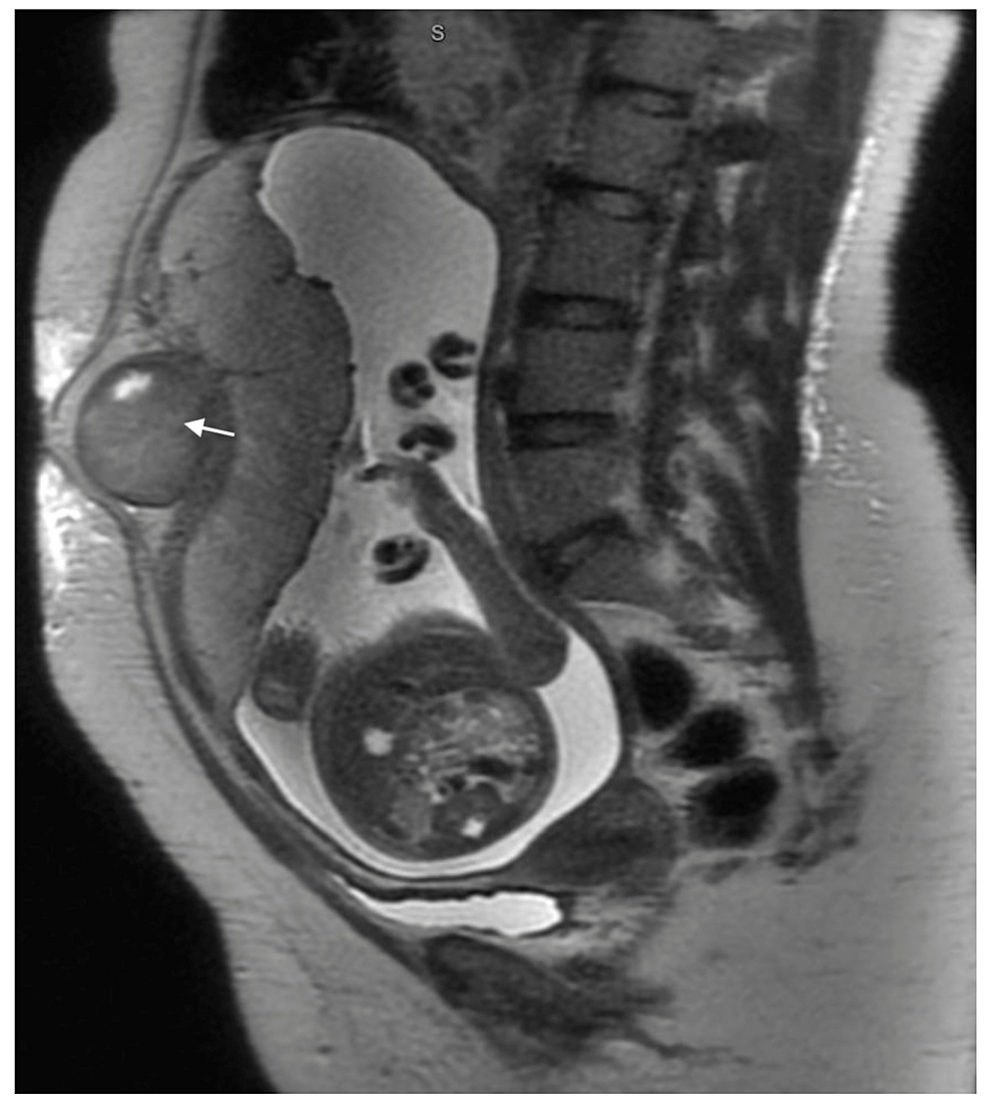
Sagittal T2 weighted MRI shows a sub-serosal fibroid with red degeneration along the anterior wall of the uterus in an upper uterine segment (white arrow)
